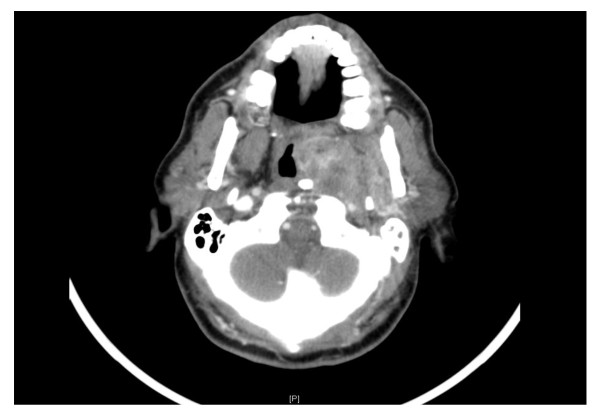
CT scan showing left parapharyngeal soft tissue mass protruding into oro and nasopharynx.
